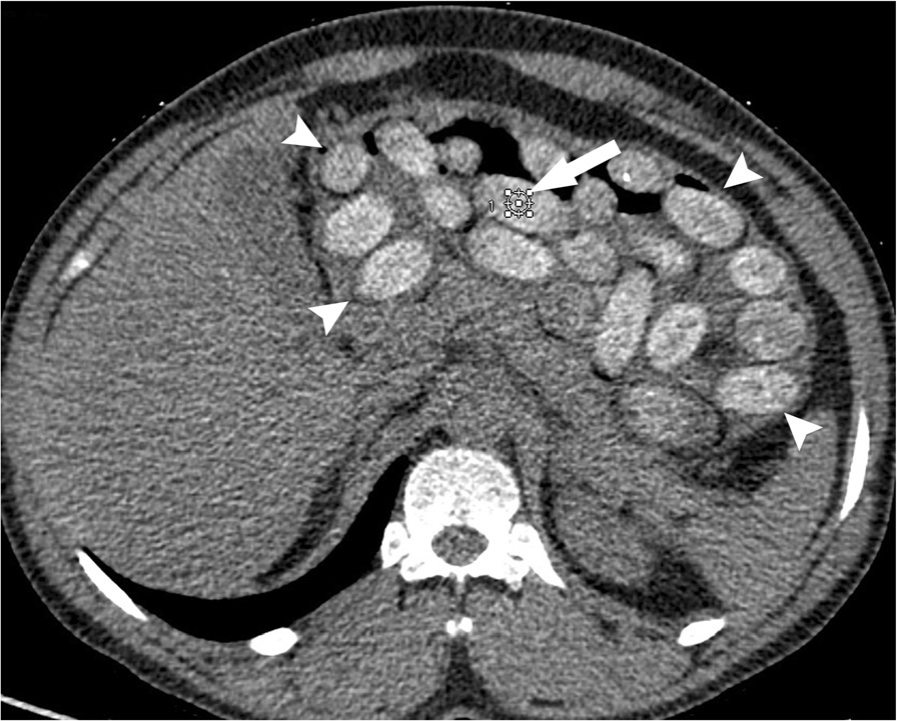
Thirty-years-old man, positive for conveying dug packets. Axial low-dose CT scan shows numerous drug containers inside the stomach (*arrowheads*); measurement of attenuation is performed within one drug container (*arrow*)
